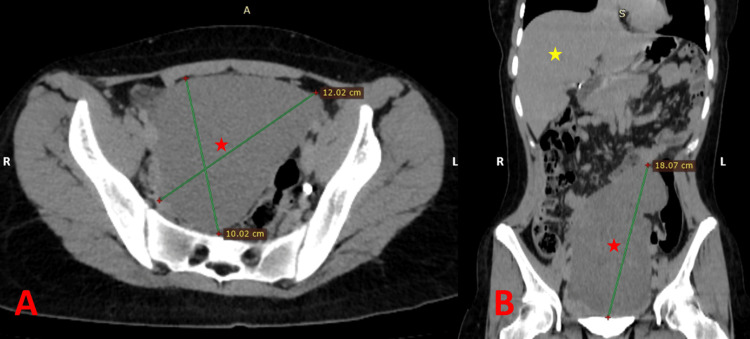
CT scan of the patient at the time of admission. A and B: CT of the abdomen and pelvis, revealing the appearance of the wandering spleen in the lower abdomen and pelvic area in the axial and coronal sections, respectively (the red star represents the spleen and the yellow star represents the liver).
